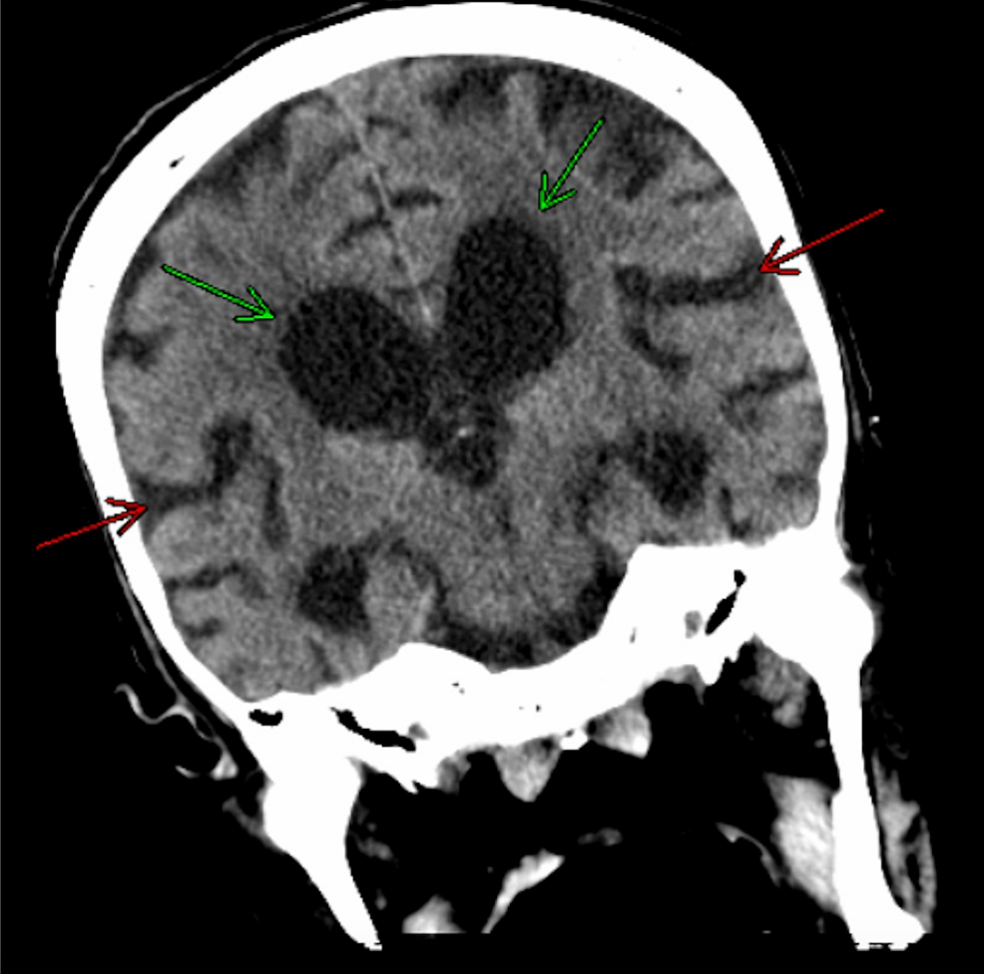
Ventriculomegaly and diffuse parenchymal volume loss Dilatation of the lateral ventricles (green arrows) and sulcal prominence (red arrows) on non-contrast CT of the head.
